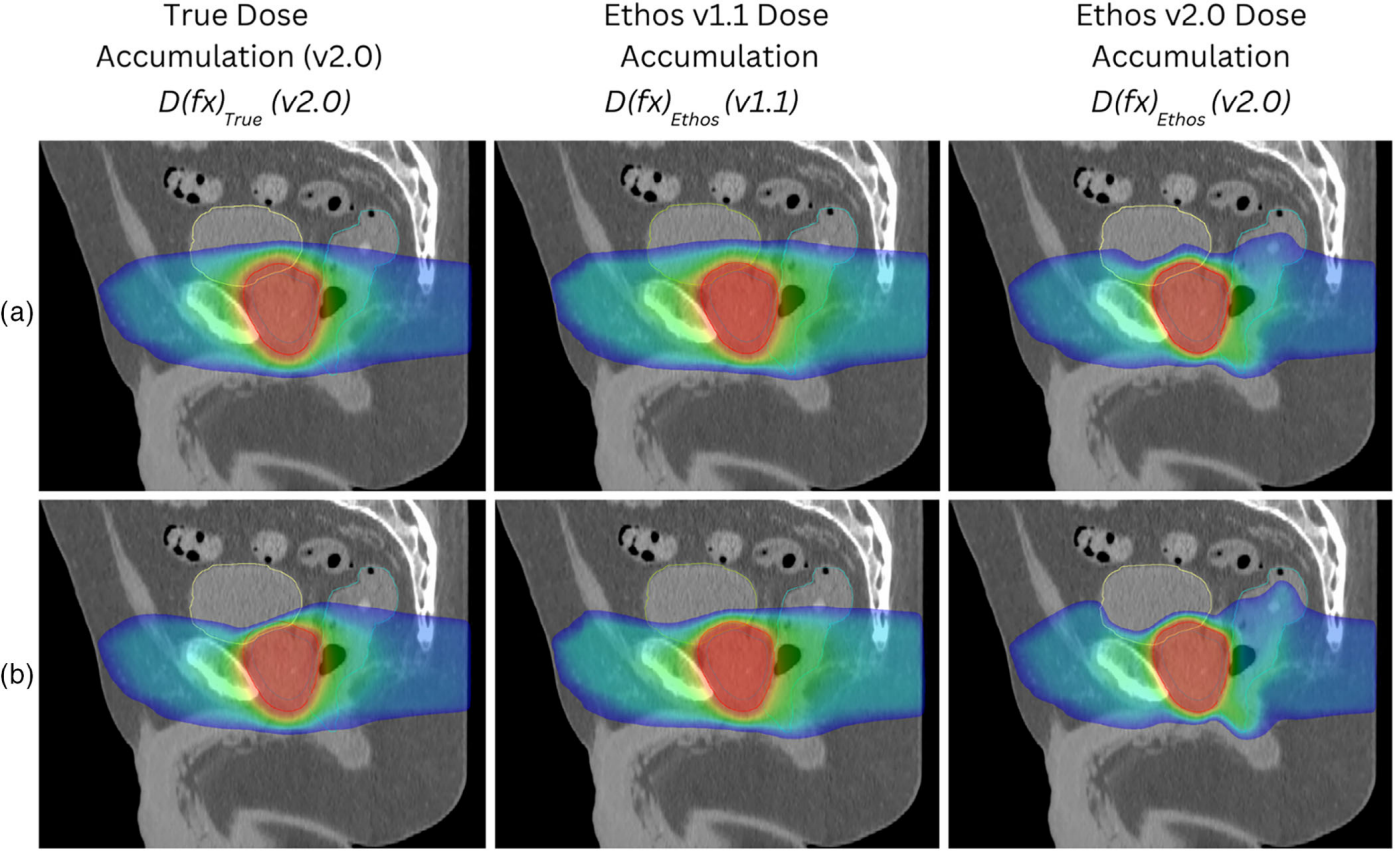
Sagittal visualization of the accumulated dose overlaid on the planning CT. Row A displays accumulated doses from *Part 2 ‐ Prostate* Fx1, where no deformation was applied to the planning CT. Row B displays accumulated doses from *Part 2 – Prostate* Fx4, where the bladder was expanded by a large amount. Note that *D(fx)_True_
